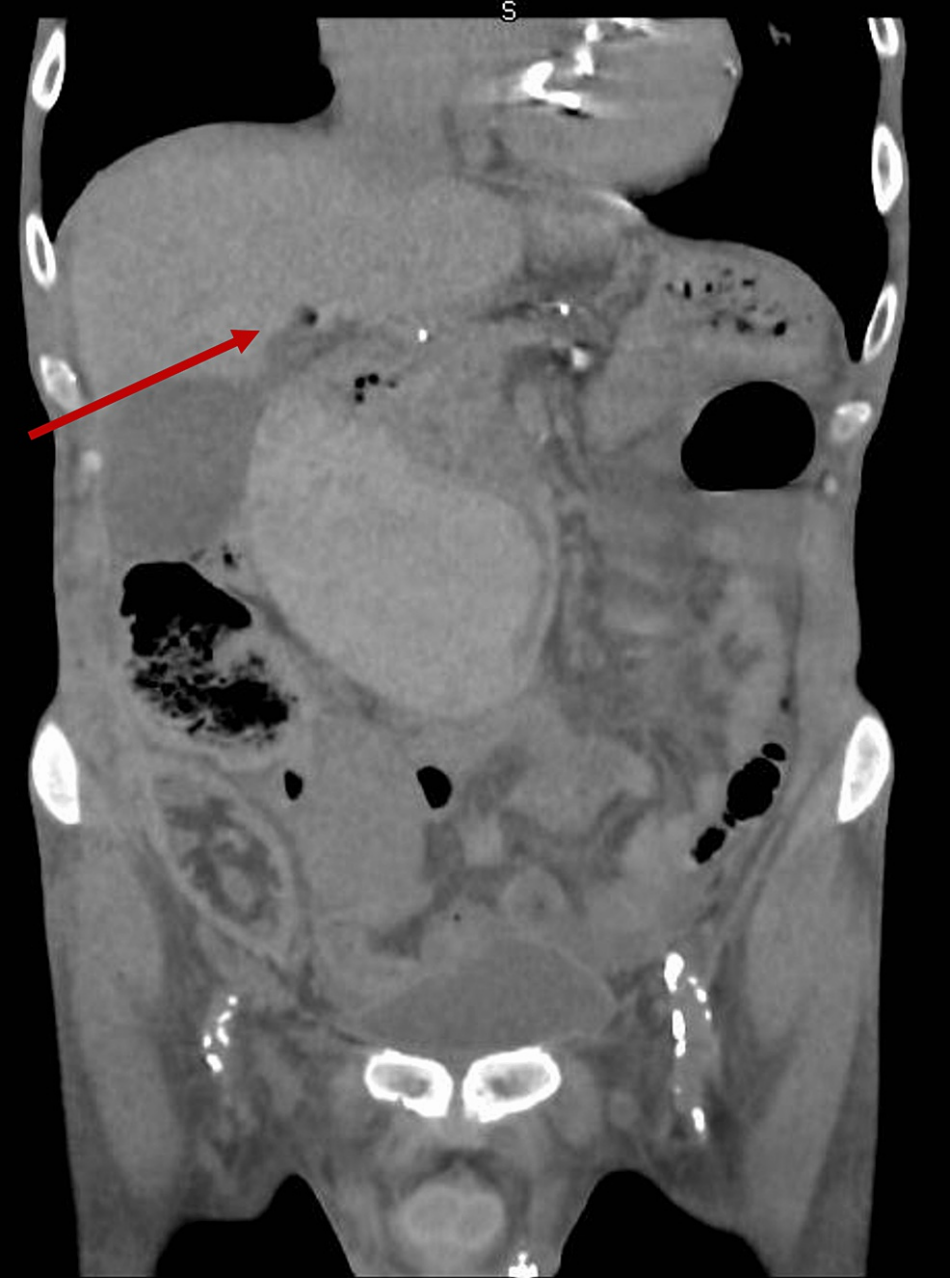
CT Abdomen/Pelvis (coronal view) The image is showing resolution of the previous HPVG (Hepatic Portal Venous Gas)
